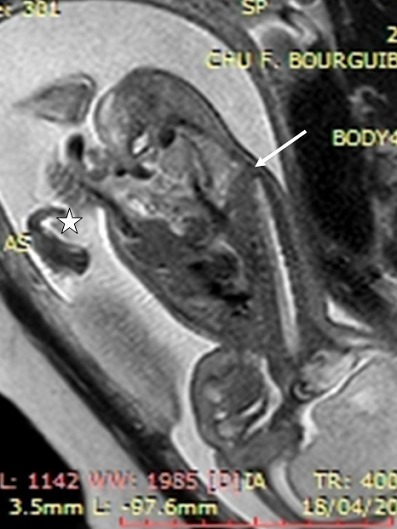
Fetal MRI in SE T2, in strict sagittal section confirming the agenesis of the lumbosacral spine (arrow); the omphalic sac is also visualized (star)
